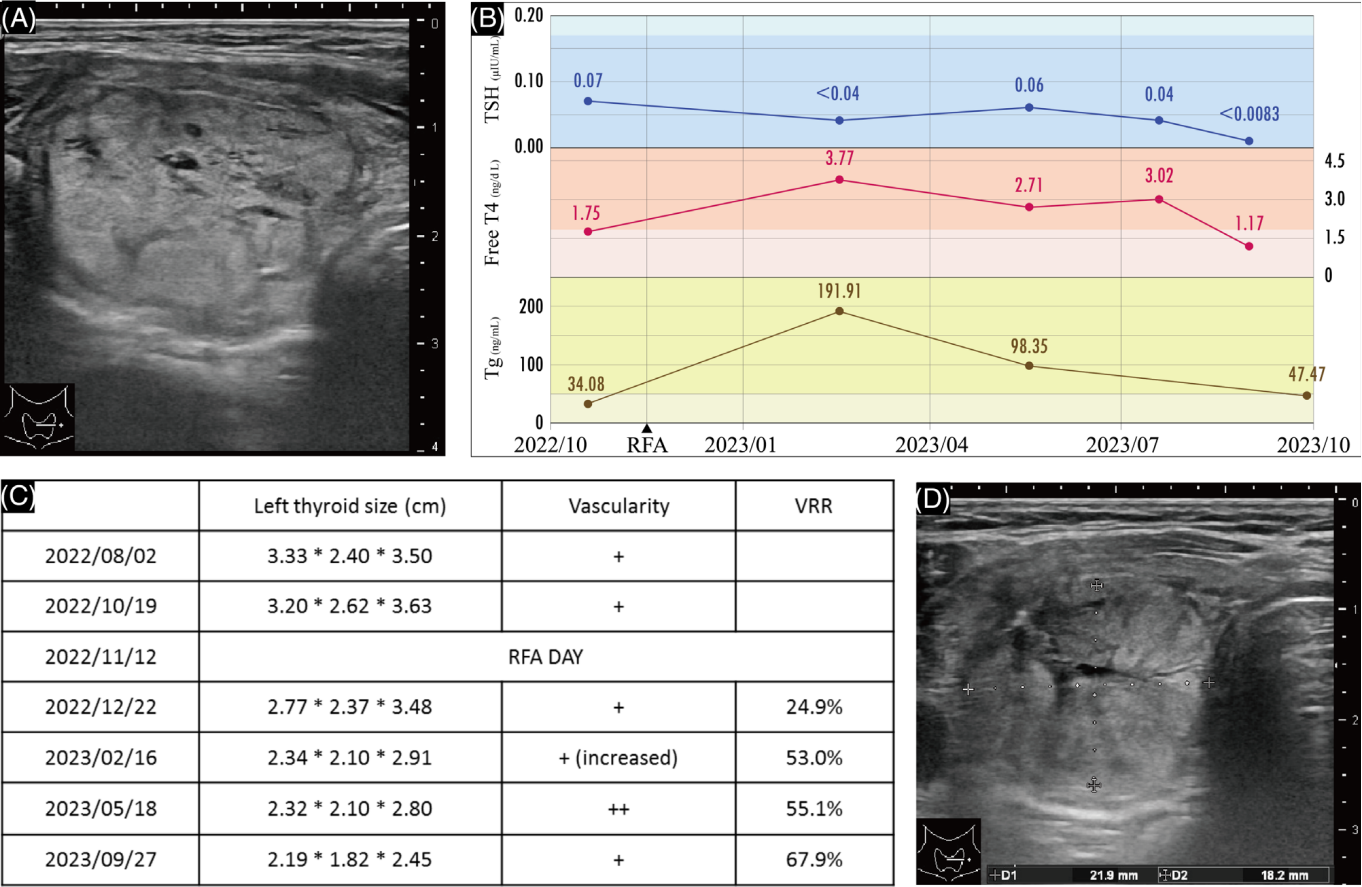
(A) Pre‐radiofrequency ablation (RFA) sonography revealed a 3.20 × 2.62 × 3.63 cm left thyroid nodule. (B) Free T4, TSH, and thyroglobulin (Tg) levels over time with reference values in lighter‐colored blocks. (C) Thyroid nodule size, vascularity, and volume reduction rate (VRR) were demonstrated. *Vascularity, “+” for peripheral, “++” for central vascularity under 50% of the volume. (D) Post‐RFA sonography at 46 weeks revealed the nodule measuring 2.19 × 1.82 × 2.45 cm.
